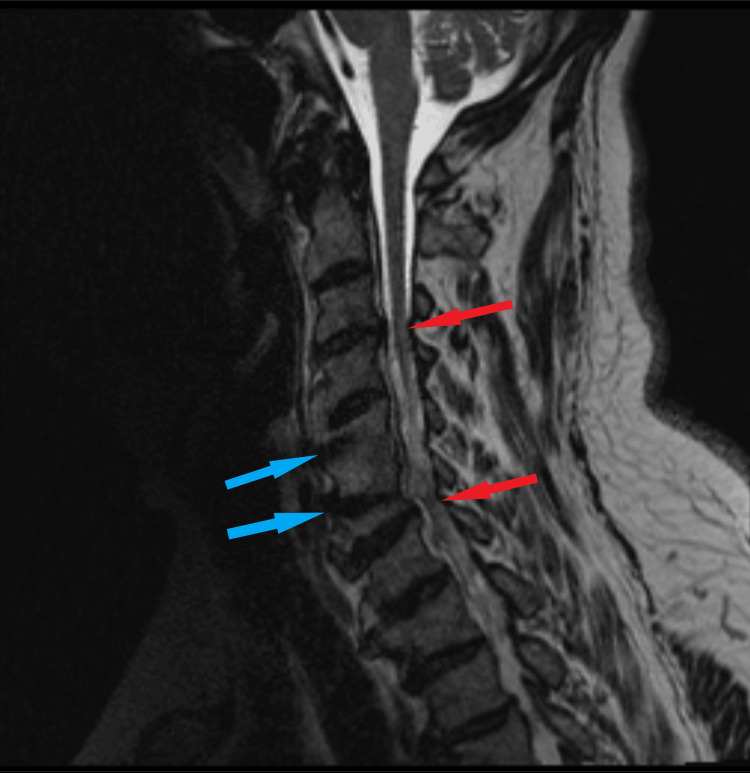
T2-weighted axial magnetic resonance imaging (MRI) of the cervical spine without contrast demonstrates multilevel chronic degenerative changes with central canal stenosis at the C3-C4 and C6-C7 levels (red arrows). Postsurgical changes from a prior anterior cervical discectomy and fusion (ACDF) procedure at the C5-C6 level with fixation hardware including vertebral screws (blue arrows) are present.
